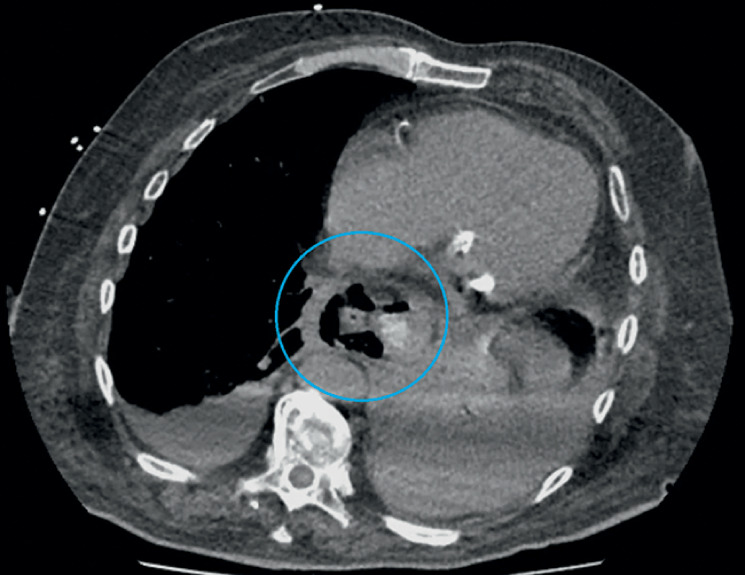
Computed tomography scan with injection and ingestion, showing a circumferential hydroaeric infiltration of the distal esophagus, with a suspected esophageal rupture (blue circle)
